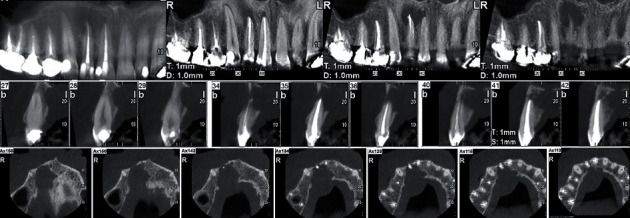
CBCT scan of the maxillary anterior region showing a large bony defect surrounding the periapex area of tooth #12, which extended from the distal aspect of the root of tooth #13 toward the mesial wall of the root of tooth #11.
